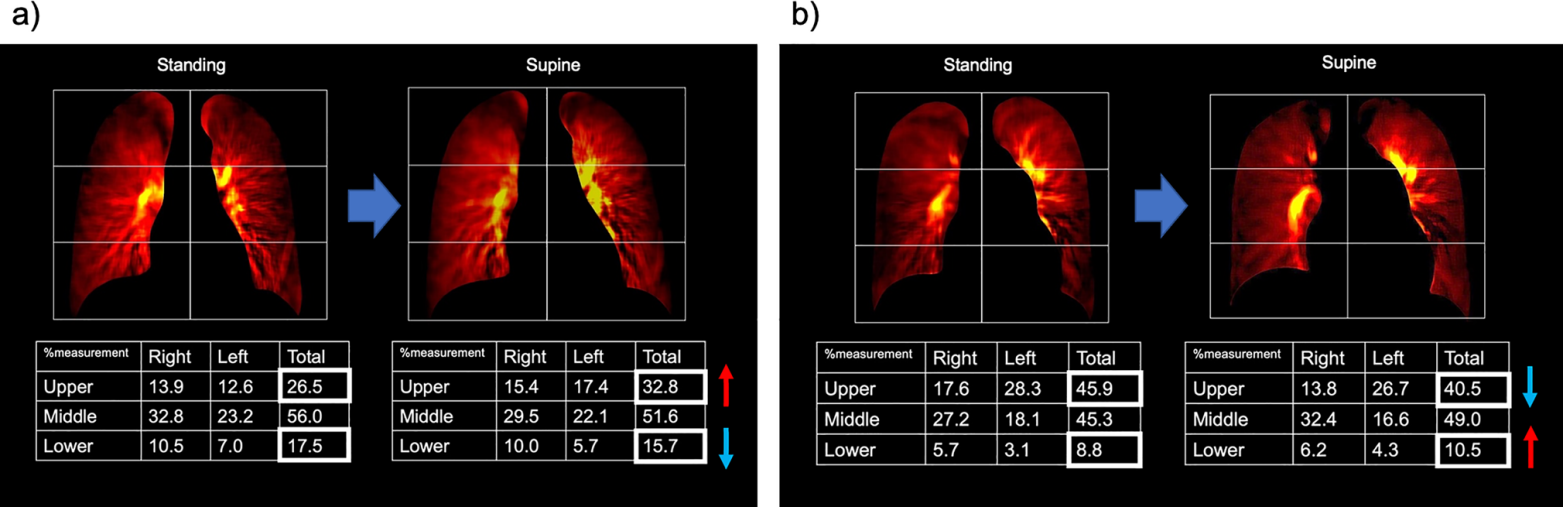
Postural changes in pulmonary perfusion assessed by the perfusion map of dynamic chest radiography in a healthy volunteer (a) and a patient with pulmonary arterial hypertension (b). With the postural change from the standing to supine position, the percentage measurement of the upper lung increased (from 26.5% to 32.8%) and that of the lower lung decreased (from 17.5% to 15.7%) in a healthy volunteer (a), whereas the percentage measurement of the upper lung decreased (from 45.9% to 40.5%) and that of the lower lung increased (from 8.8% to 10.5%) in a patient with pulmonary arterial hypertension (b)
